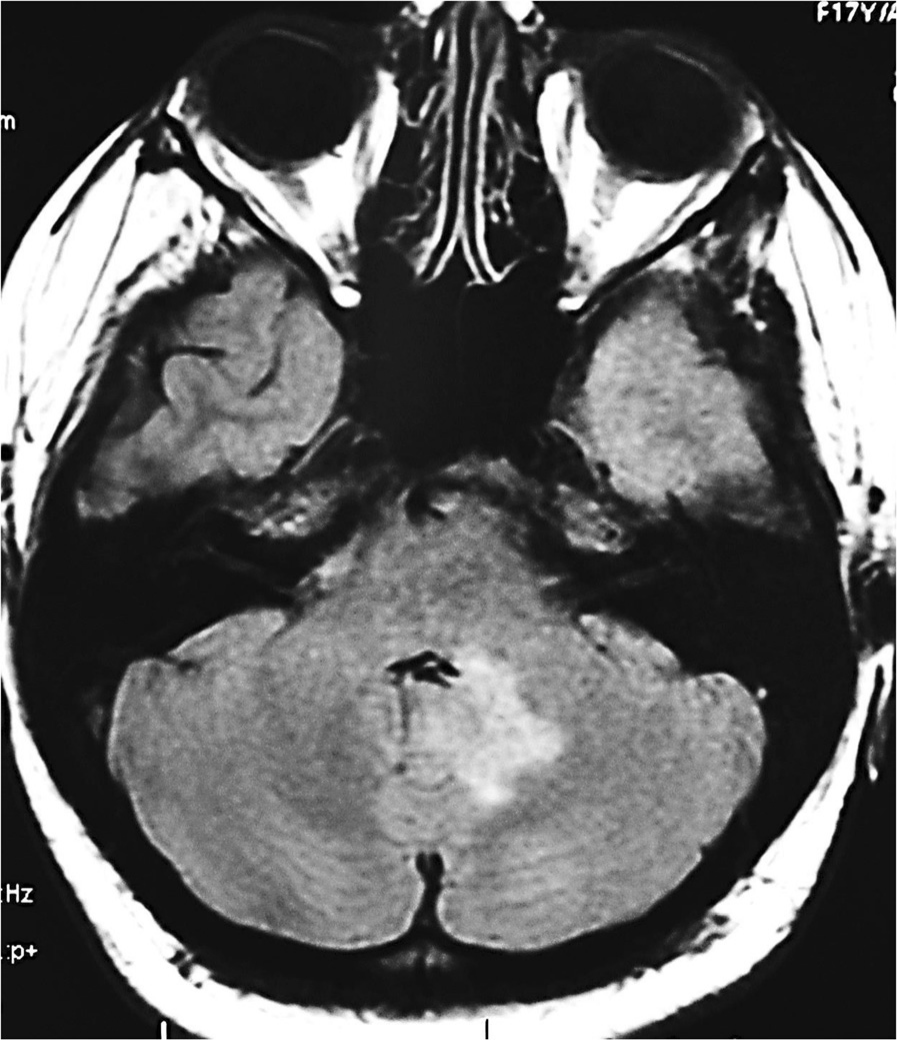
T2-weighted FLAIR magnetic resonance imaging showing high signal intensity lesion in the left cerebellar hemisphere extending into the middle cerebellar peduncle and left dorsal pons.
